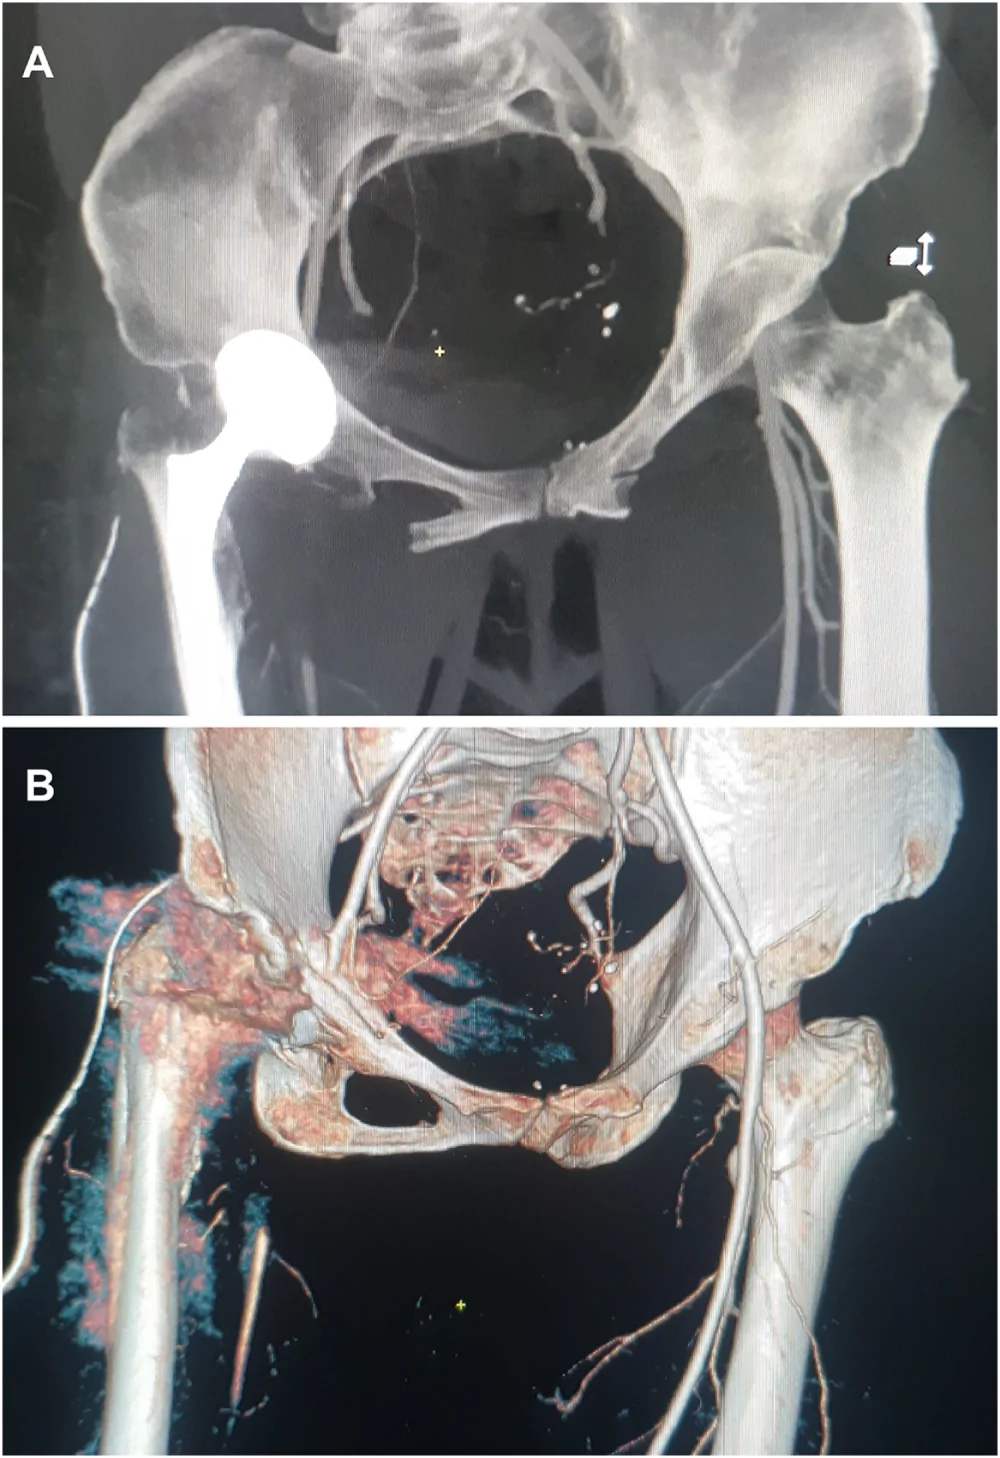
Coronal view (A) and 3D reconstruction (B) from CT angiography showing the absence of opacification in the common femoral artery at the level of the anteroinferior wall of the acetabulum, extending over 100 mm in the proximal third of the superficial femoral artery.
